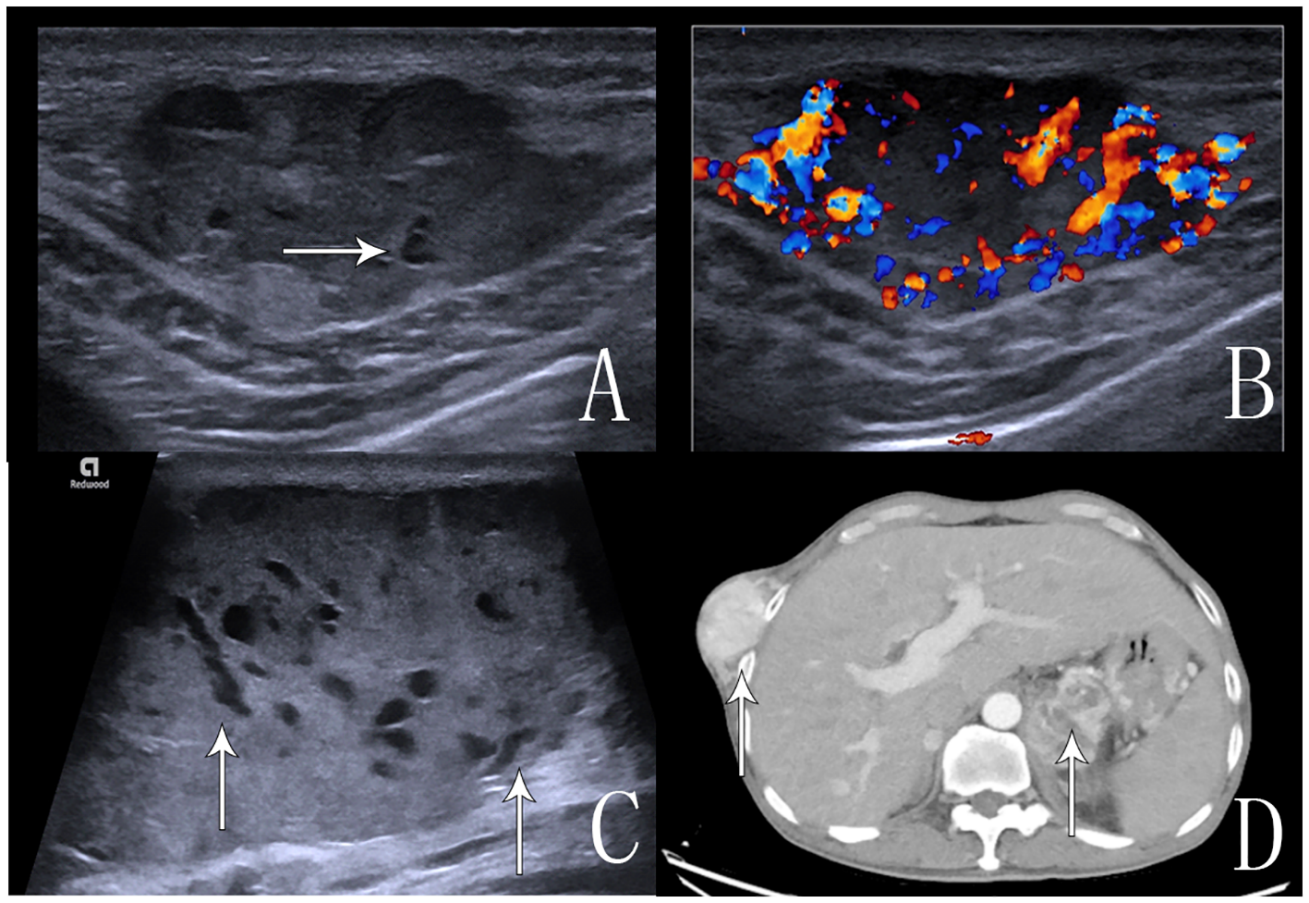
Ultrasound and CT images of the abdominal wall and chest wall masses. (A) An ultrasound image that shows anechoic echogenicity in the hyperechoic mass in the right abdominal wall. (B) An ultrasound image that shows abundant blood flow signals in the mass in CDFI. (C) An ultrasound image that shows multiple anechoic echoes in the hyperechoic mass in the right chest wall, which is a “fissure”-like alteration. (D) An enhanced CT scan, the arrow shows inhomogeneous enhancement of the right chest wall mass and left kidney tumor.
